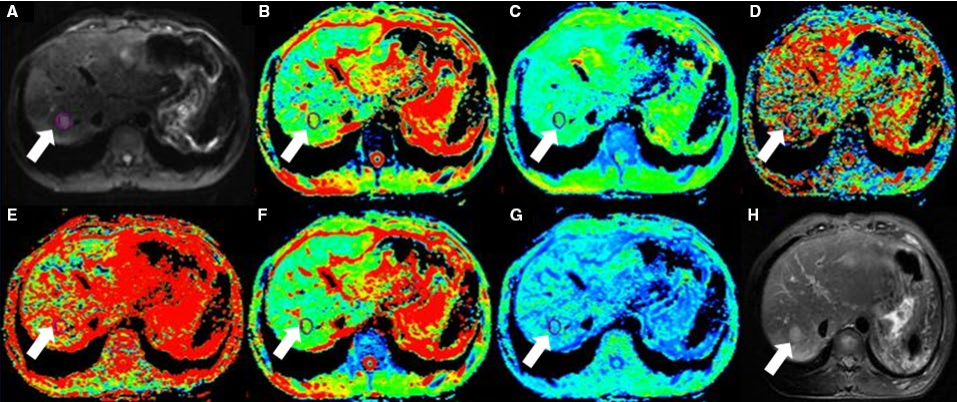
Fifty‐six‐year‐old male with HCC(arrow). (A) is b value of 50 s/mm^2^ of DWI, and (B‐G) are pseudocolor of ADC,* D*
